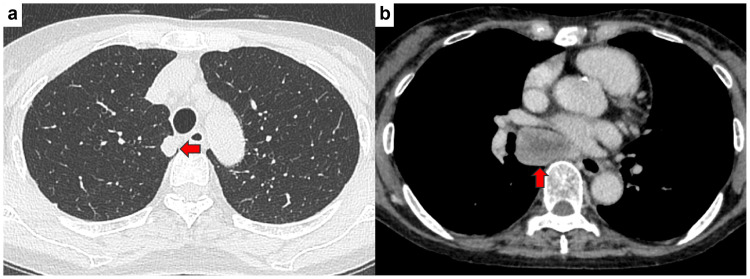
CT findings at the initial and last visits for Case 3. (a) A 16 × 12 mm nodule was found in the right upper lobe. The arrow denotes the nodule. (b) Lymph node enlargement was observed at the tracheal bifurcation, which suggested metastasis. The arrow denotes lymph node enlargement.
